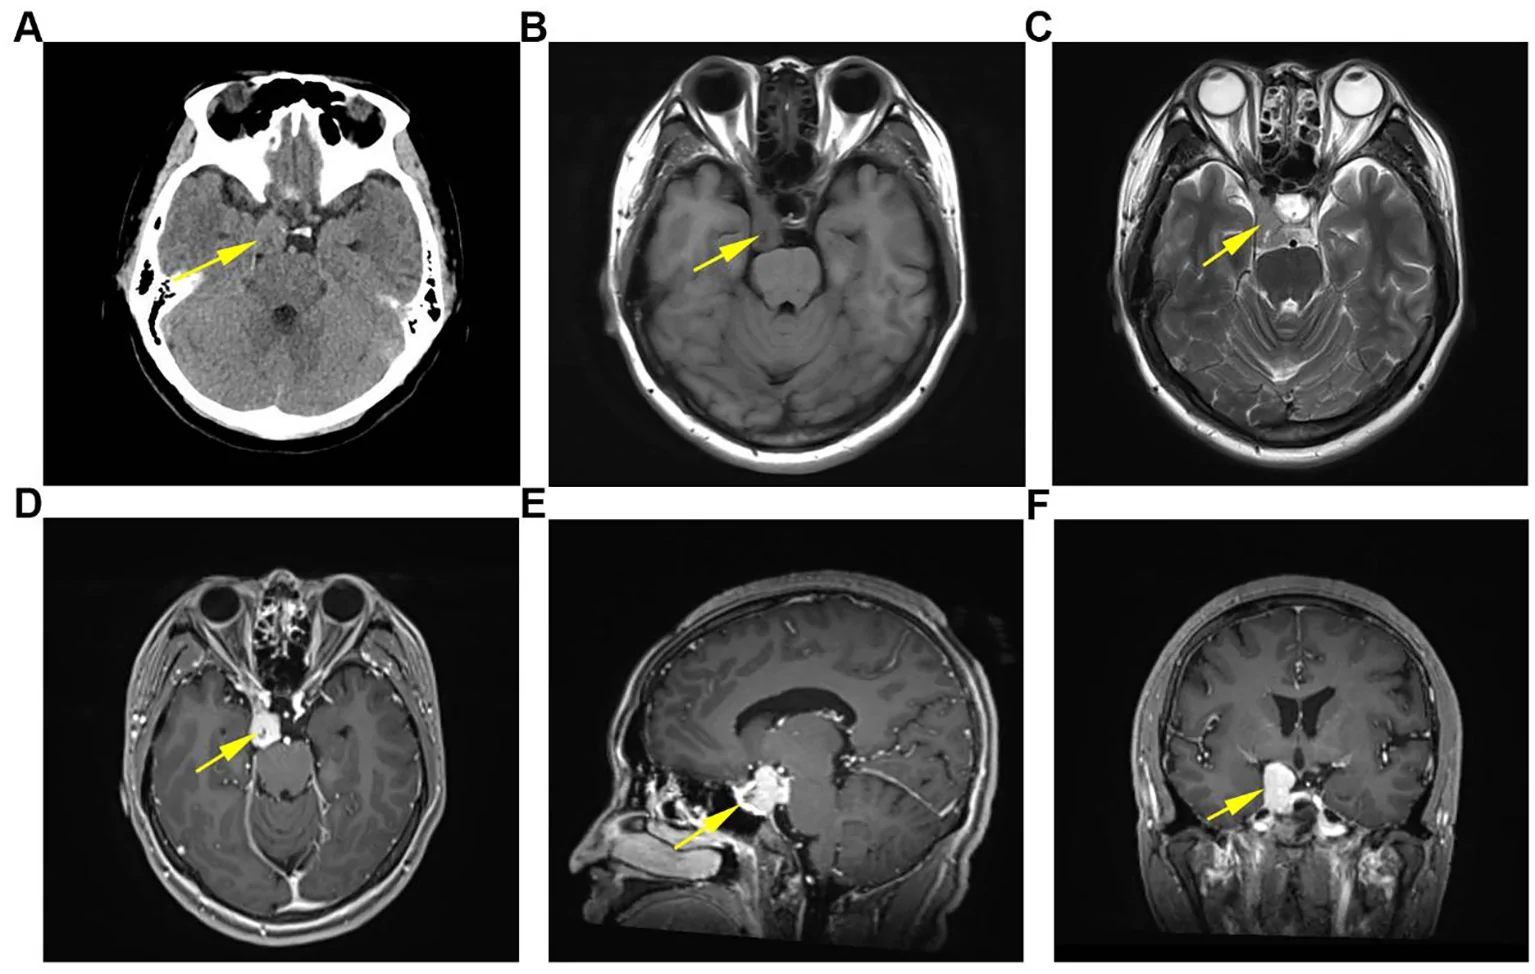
Preoperative imaging. (A) Axial non-contrast CT (brain window). (B) Axial T1WI. (C) Axial T2WI. (D) Axial CE-T1WI. (E) Sagittal CE-T1WI. (F) Coronal CE-T1WI.
